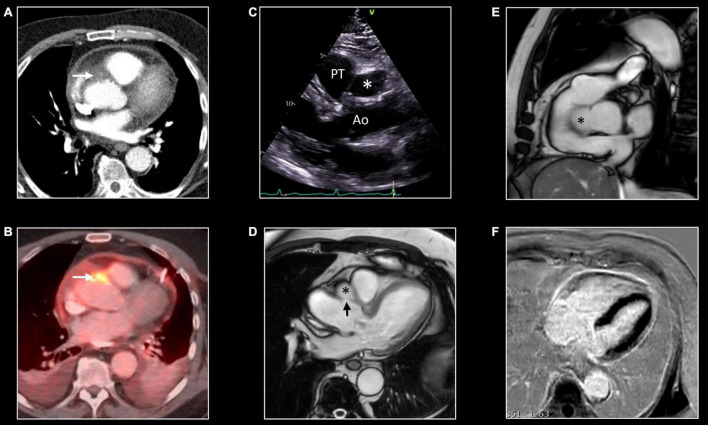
False aneurysm of the thoracic aorta. Eighty-one-year-old man with MSSA sepsis and infectious pericarditis complicated by false aneurysm formation of the thoracic aorta. CT and PET/CT performed after pericardial drainage showed thickening of the pericardial layers with enhancement at chest CT (A) and FDG uptake at PET/CT. Note the presence of a focus of strong FDG uptake [arrow, (B)] with infiltration of the epicardial fat [arrow, (A)] at CT. These findings were interpreted as inflammation of one of the pericardial sinuses. However, follow-up transthoracic echocardiography, 3 months later, showed echo-lucent structure [*,(C)] between the aorta (Ao) and pulmonary trunk (PT). CMR shows the presence of a small pseudo-aneurysm [*,(D,E)] in communication with the thoracic aorta at the level of the sinotubular junction [arrow, (D)]. Note the residual enhancement of the pericardial layers at LGE imaging, (F) reflecting residual pericardial inflammation.
